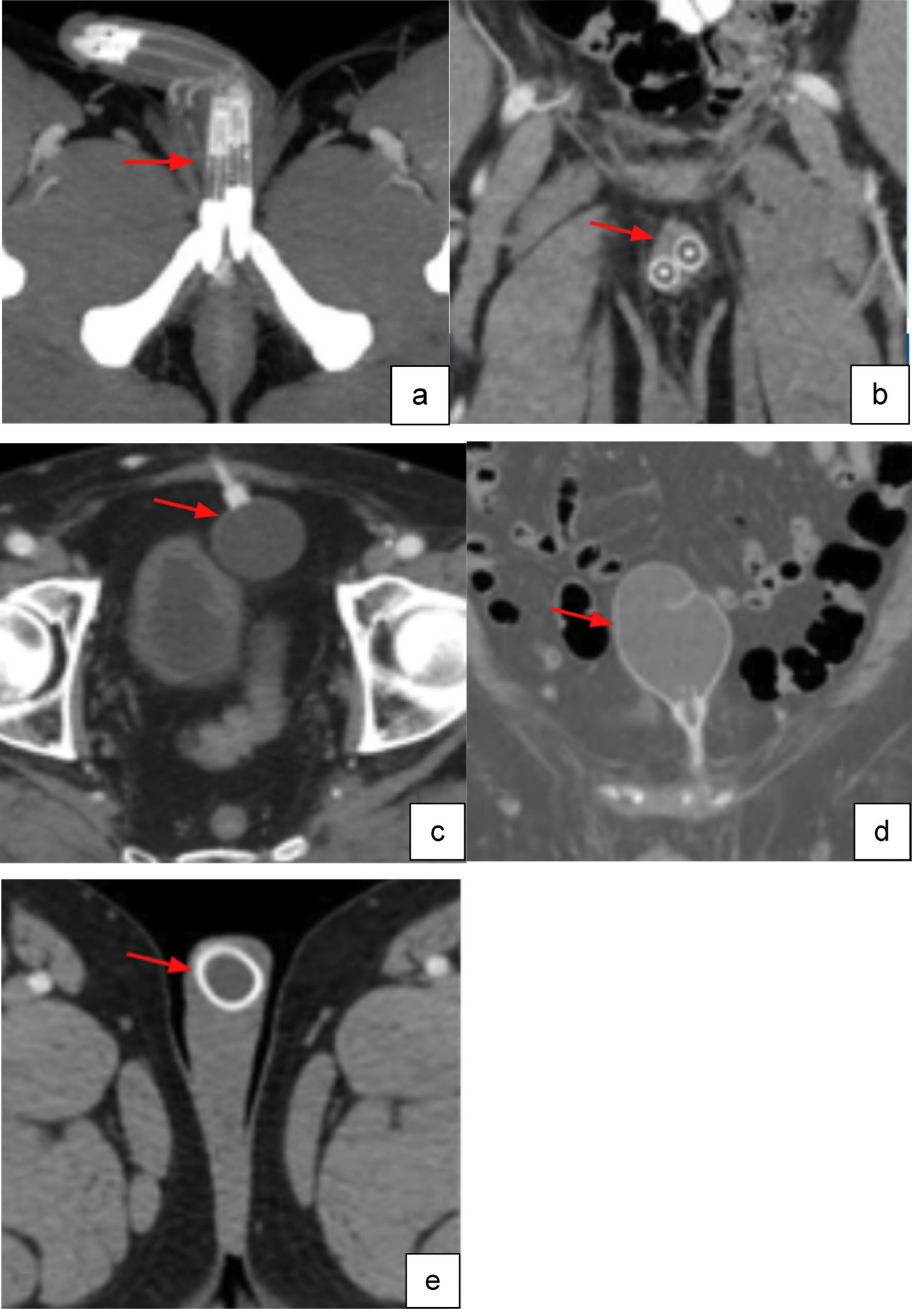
Axial (a) and coronal (b) CT images with IV contrast of normal three-piece PP paired inflatable cylinders in corpora cavernosa (red arrows). Axial (c) and coronal (d) CT images of normal PP abdominal placement of reservoir (red arrows). Axial (e) CT image of normal PP pump location in the midline inferior scrotum (red arrow)
